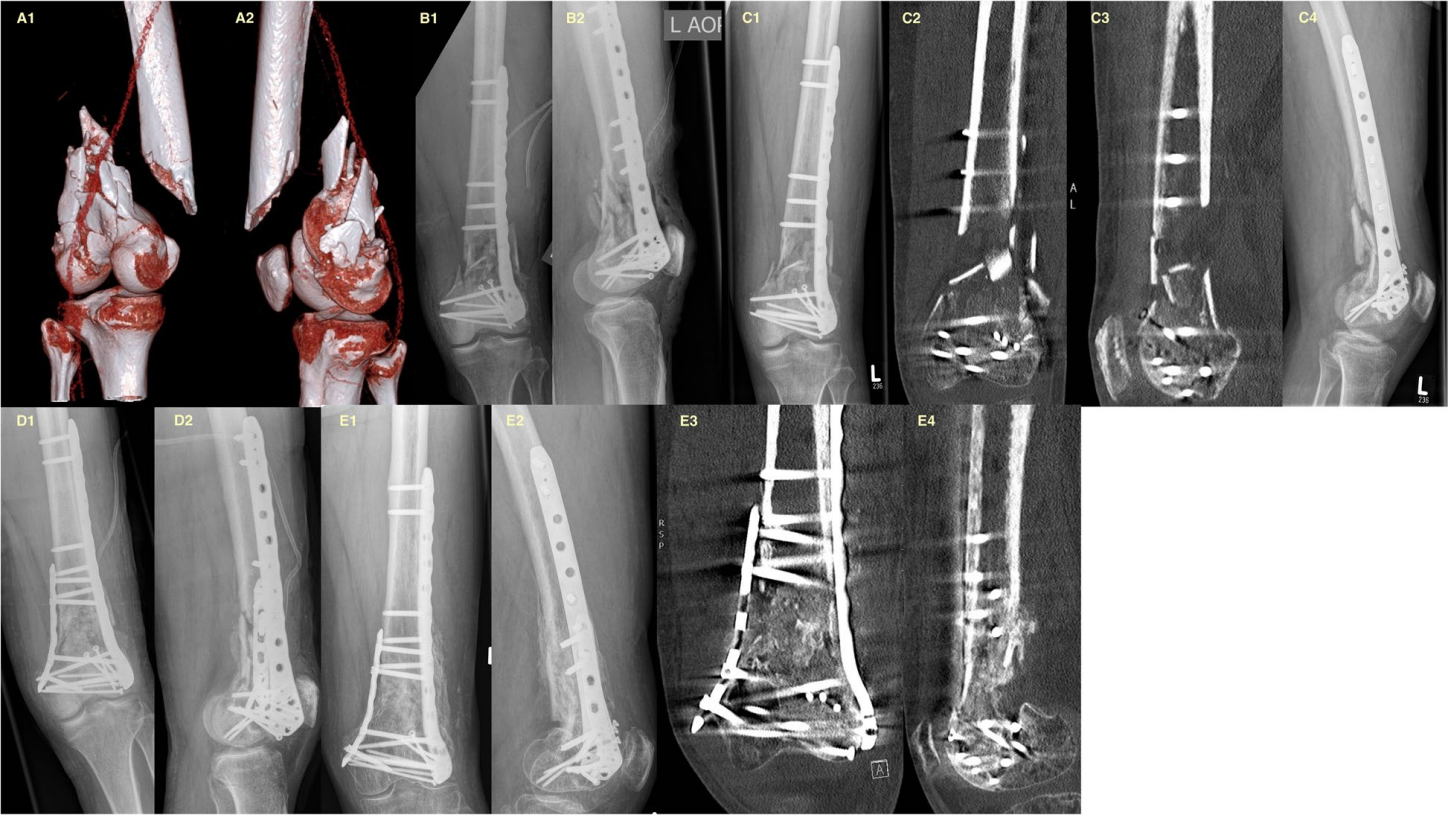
A Initial CT scan of an OTA type 33 – C2.3 distal femoral fracture of a 55-year-old male after a motorcycle accident. B X-rays showing ORIF with a lateral locking plate. C Nonunion six months after initial treatment. D Postoperative X-rays after revision utilizing a medial augmentation plate. E Final X-rays of the same patient showing bony healing six months after revision
